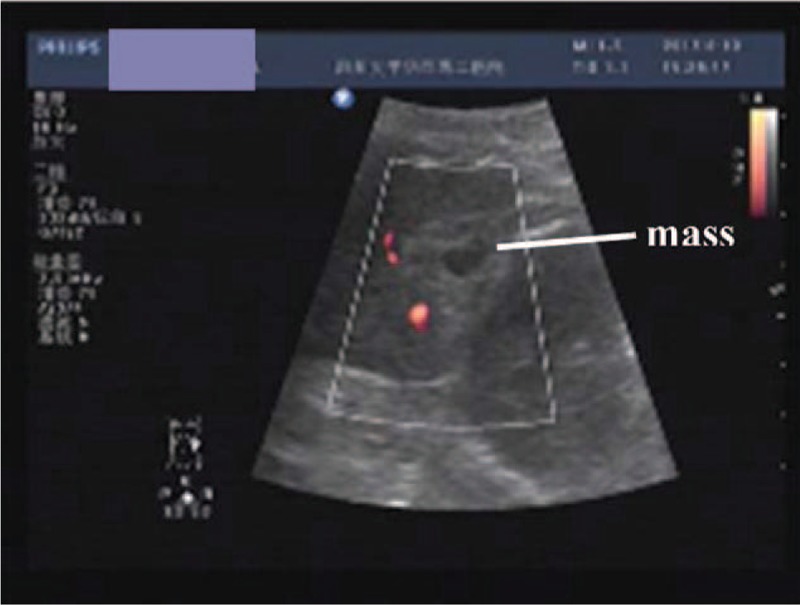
Abdominal ultrasound showing the presence of a well-defined heterogeneous a lightly hyperechogenic mass with anechoic area (0.9 cm in diameter) inside at the lower splenic pole.
